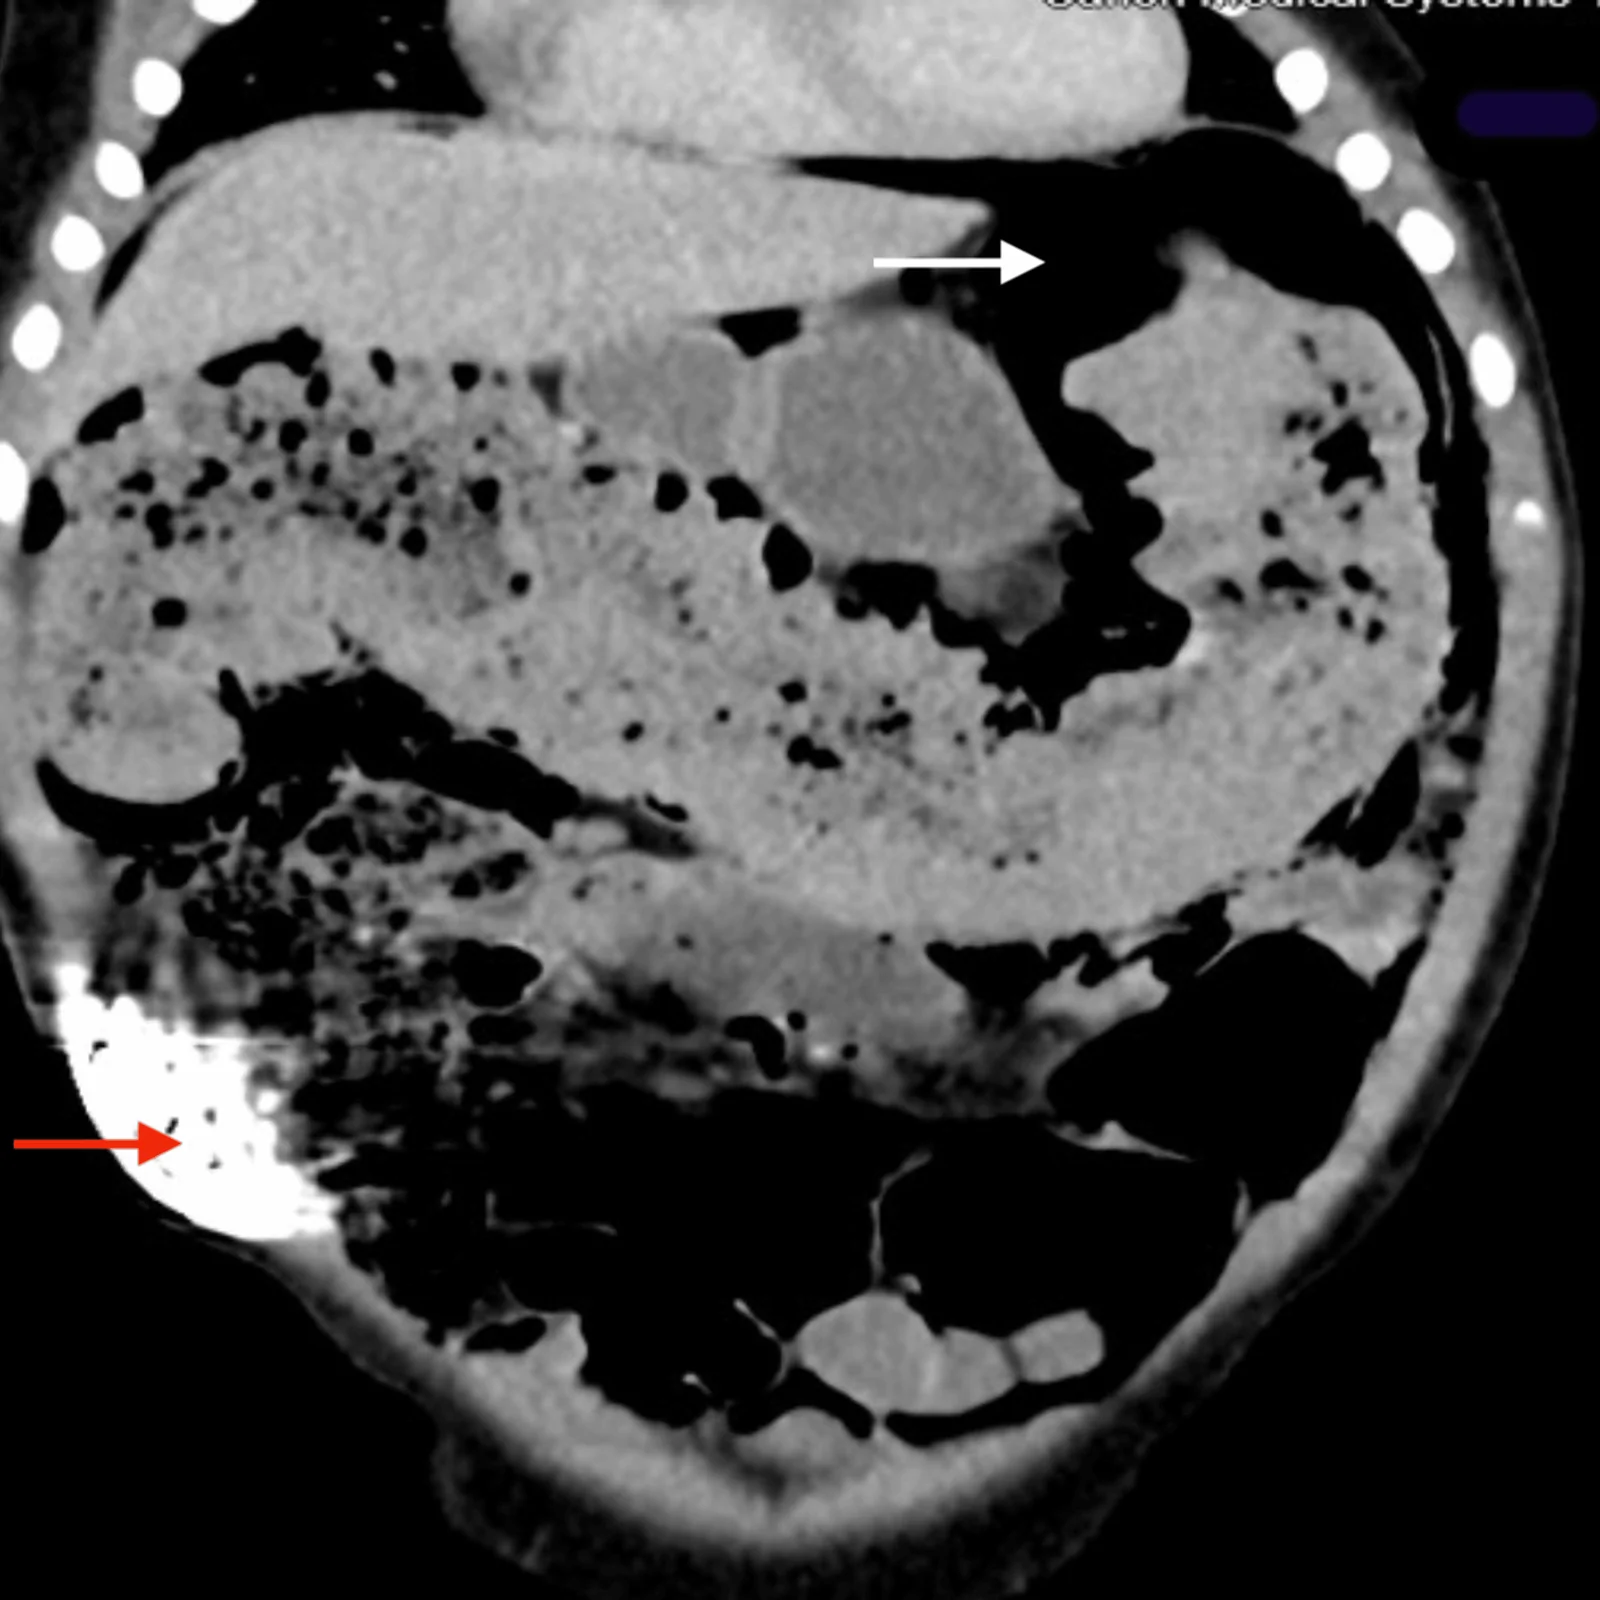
CT scan abdomen Coronal section showing large pneumoperitoneum (white arrow), diffusely dilated loops of the small and large bowel filled with faeces, pneumatosis coli, deep brain stimulator in place (red arrow), and no free fluid. The remainder of the abdominal and pelvic organs and osseous structures appear normal.
